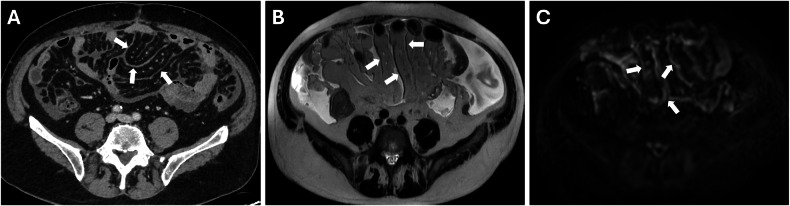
Mesenteric root infiltration in a 54-year-old man with colon cancer. CT portal venous phase (A), axial plane, the “misty mesentery” pattern with edema alongside the vessels (arrows). This pattern was confirmed also on MRI T2w (B, arrows) and on DWI (b = 800 mm^2^/s) (C, arrows)
